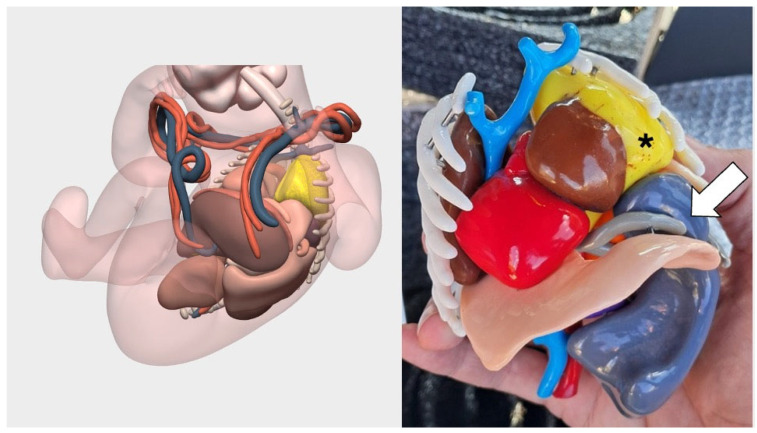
Case of left-sided diaphragmatic hernia with congenital pulmonary airway malformation (CPAM). Virtual reconstruction based on fetal MRI and 1:1 scale printed model. The CPAM is in yellow and marked with asterisk. The white arrow indicates the diaphragmatic defect.
